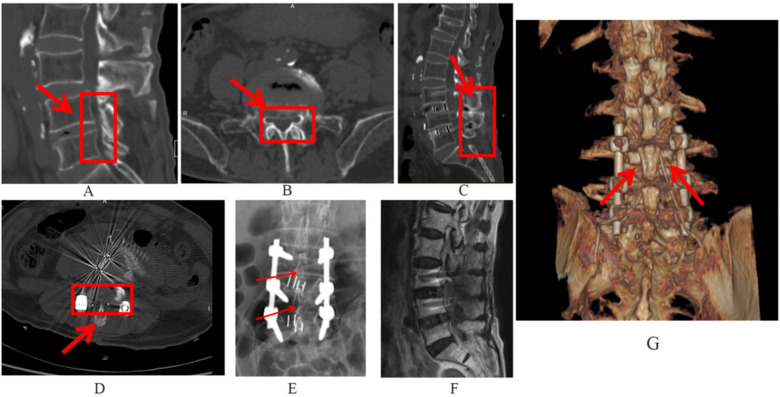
Preoperative and postoperative imaging of two-level lumbar spinal stenosis treated with bilateral laminectomy and fusion (BLF) from a representative 58-year-old male patient. (B) Axial preoperative computerized tomography (CT) demonstrating a trefoil-shaped canal and lateral recess stenosis. (C) Sagittal postoperative CT showing preservation of the spinous processes. (D) Axial postoperative CT demonstrating bilateral laminectomy. (E, F) Postoperative radiographs showing internal fixation and adequate decompression of the dural sac. (G) Three-dimensional CT reconstruction demonstrating the extent of bilateral laminectomy.
